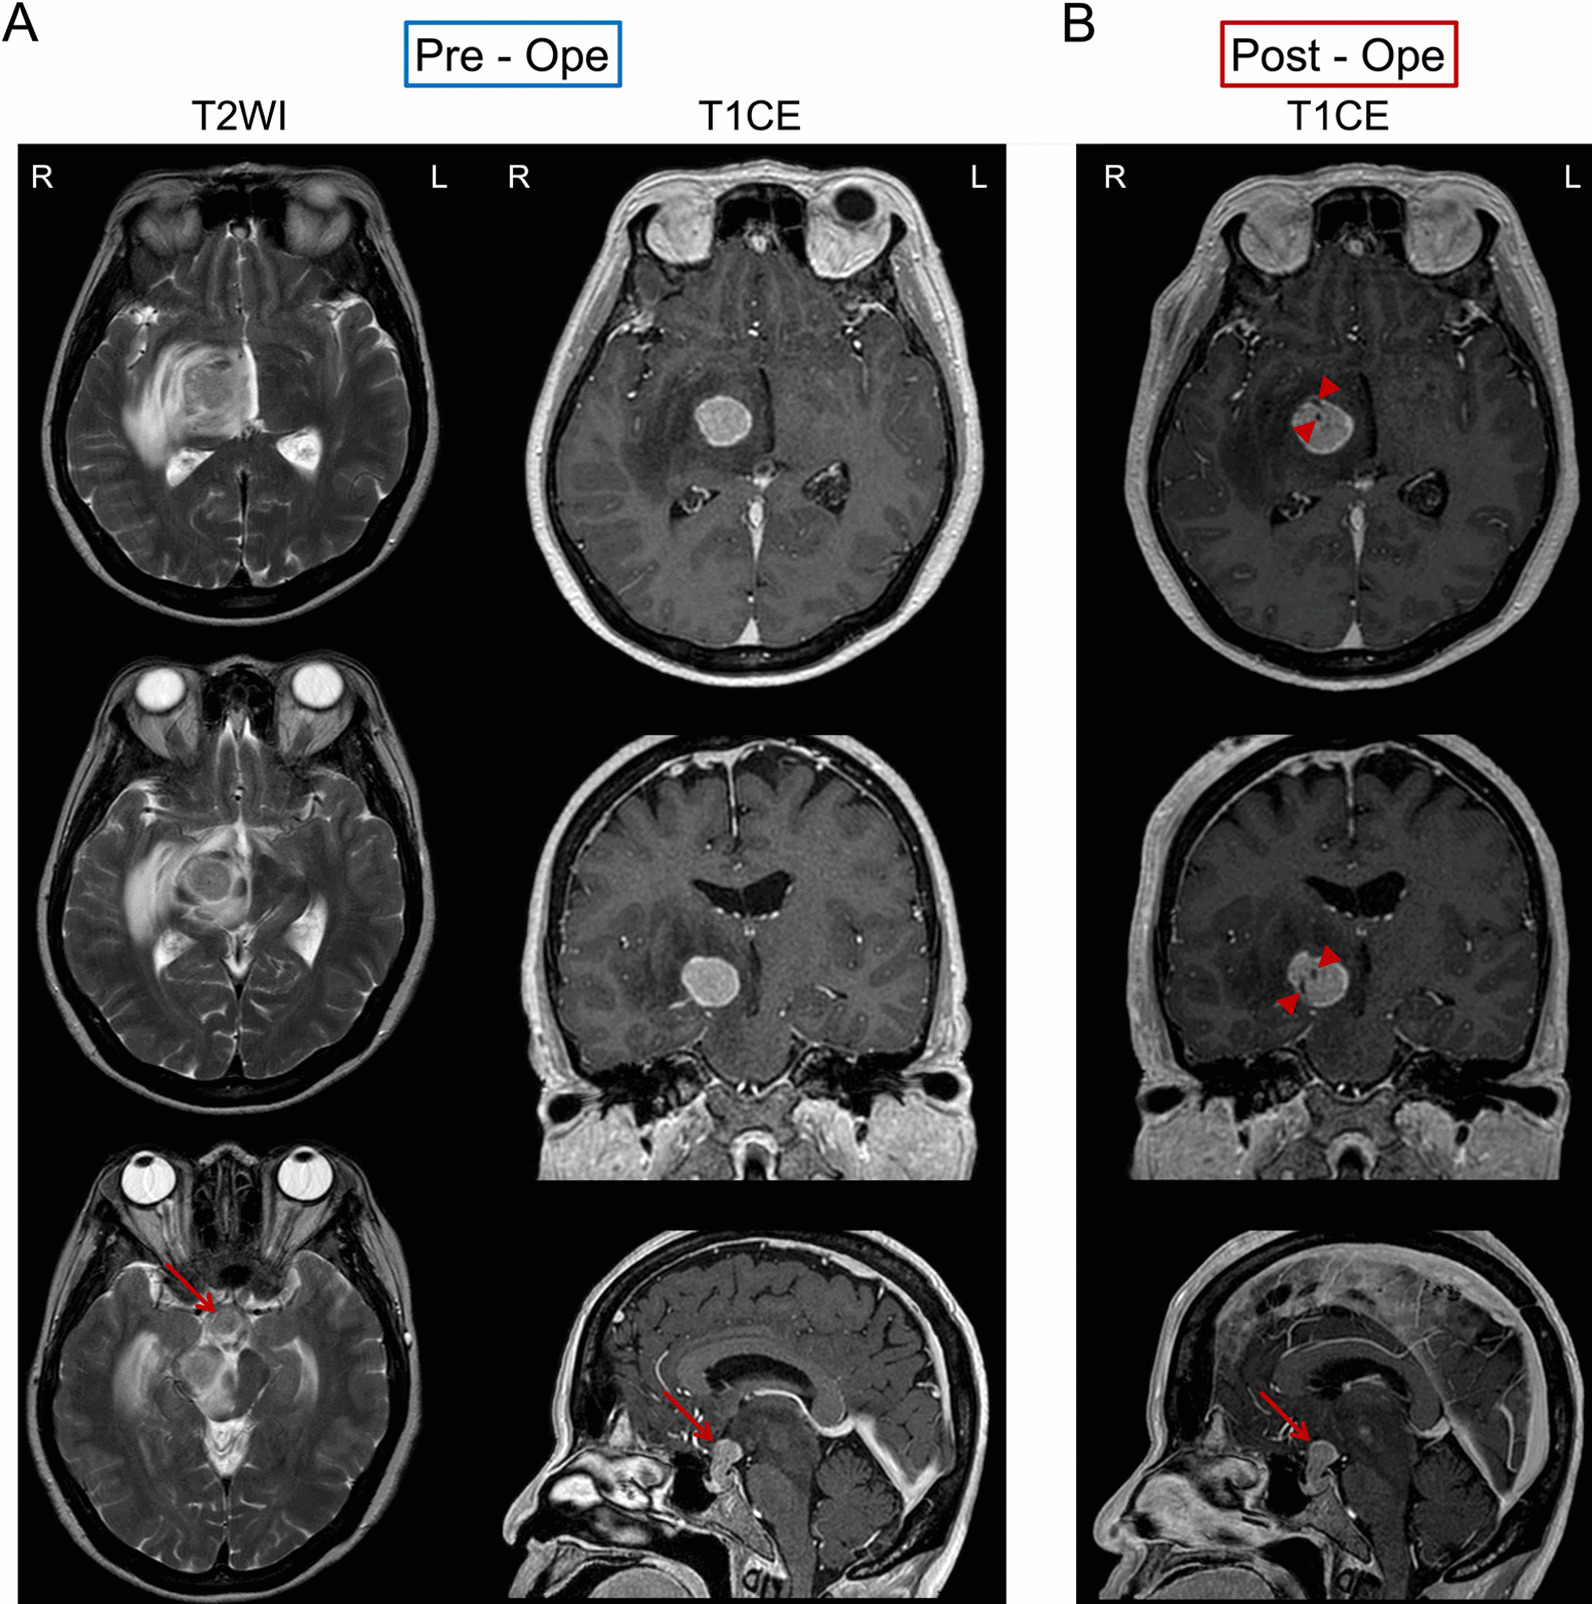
Pre- and postoperative MRI images. A High-density areas on T2WI (left column) are observed around the right thalamus lesion, with invasion of the right pedunculus cerebri. Lesions in the right thalamus and hypothalamic–pituitary axis have been shown on contrast-enhanced T1WI (right column). There is thickening of the pituitary stalk. B Postbiopsy contrast-enhanced T1WI targeting the right thalamus demonstrates the correct biopsy (scar due to biopsy indicated by red wedge arrows). Hypothalamic–pituitary axis lesions are indicated by red arrows
